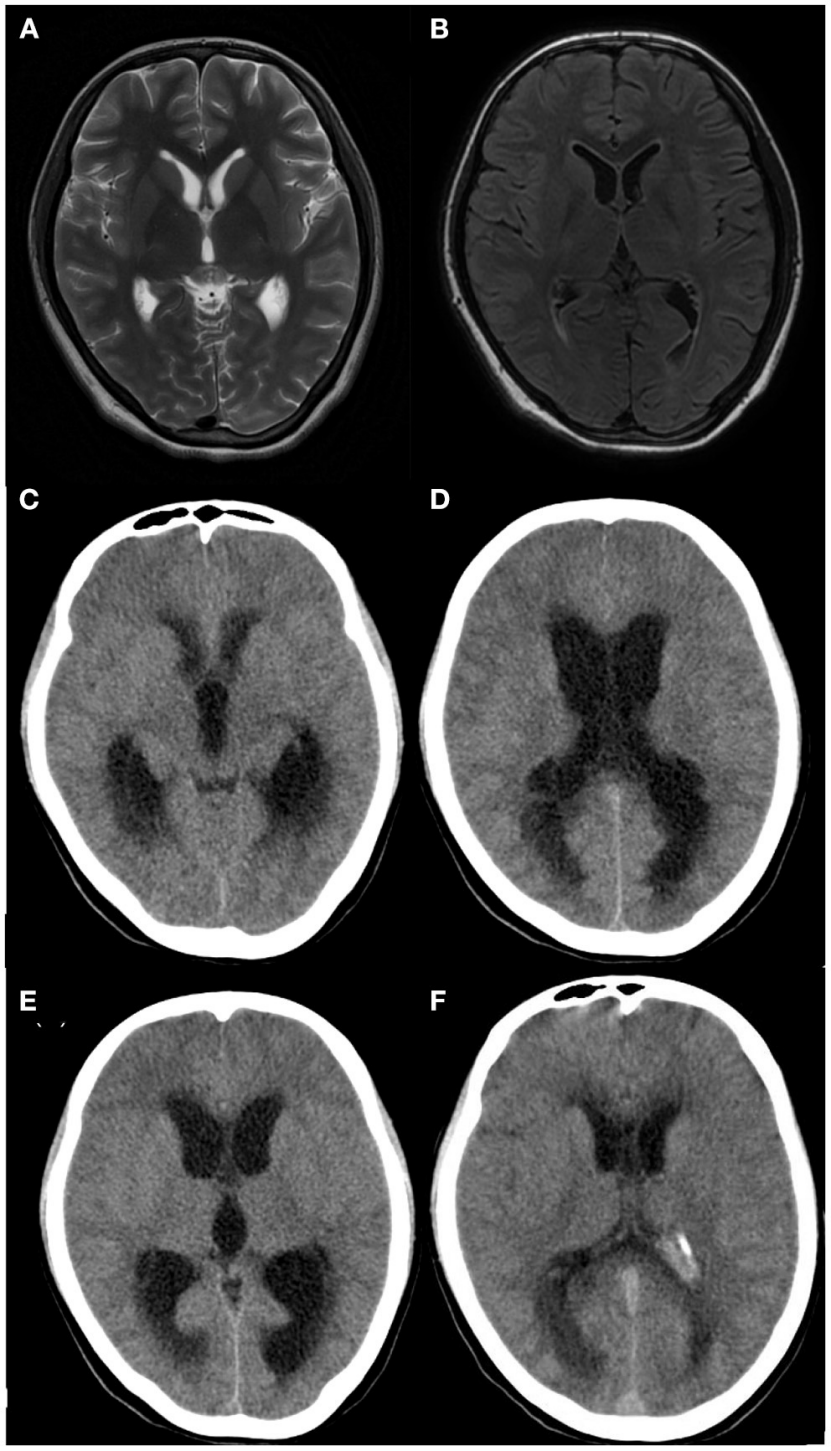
Brain imaging of patient 8. (A,B) Brain MRI performed 16 days after clinical onset showing no apparent abnormalities, (C,D) Brain CT performed 20 days after clinical onset showing hydrocephalus and parenchymal edema, (E,F) Brain CT performed after ventricular-puncture drainage (27 days after clinical onset), Though no significant reduction of hydrocephalus was shown in panel E compared to panel C, decreased hydrocephalus was obvious in panel F compared to panel D.
